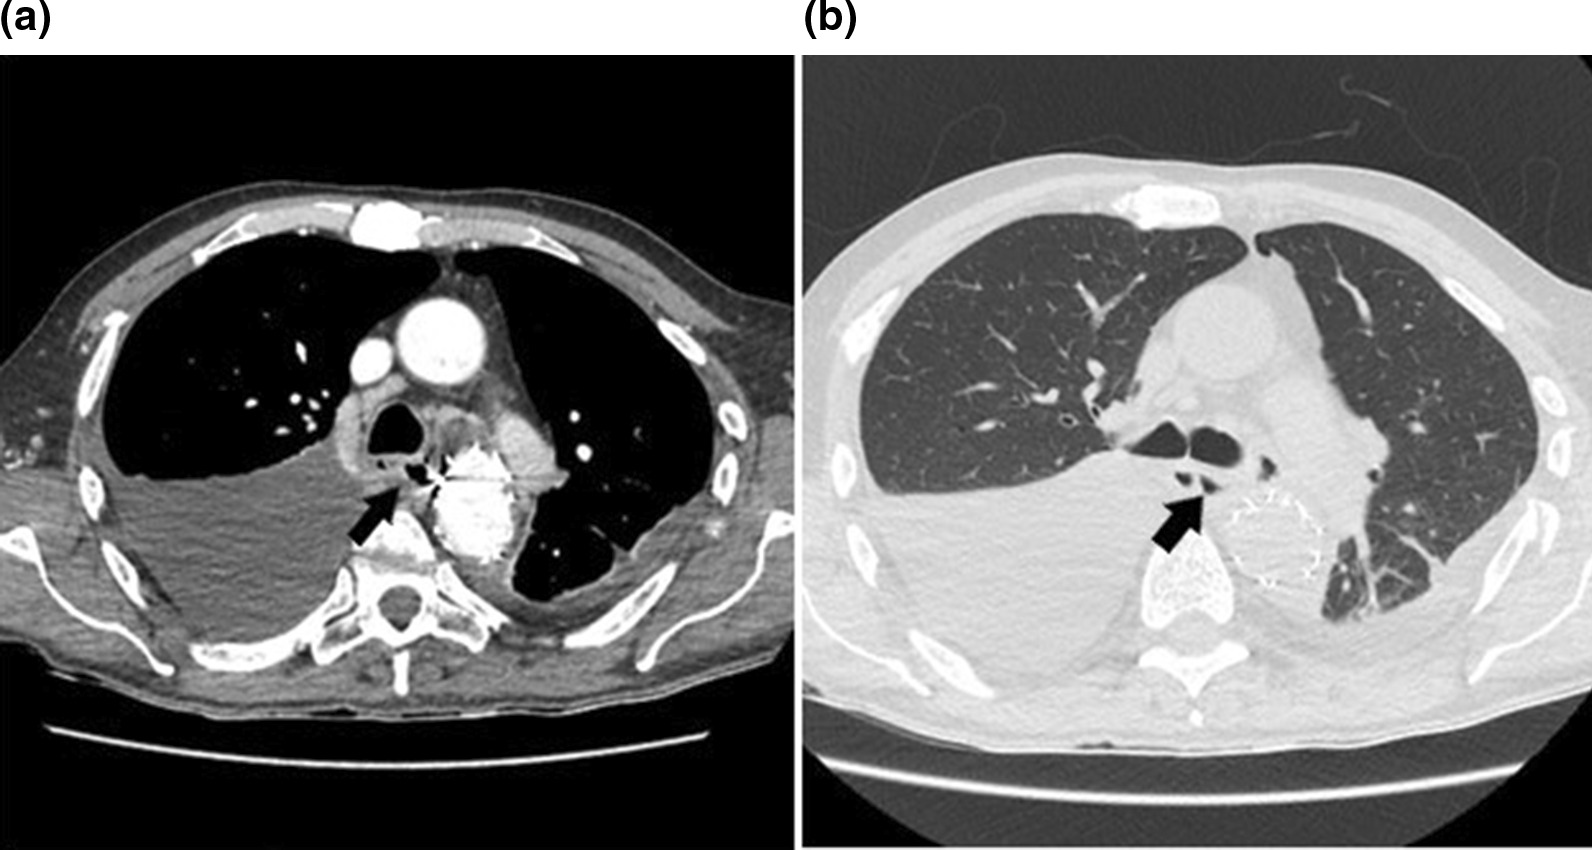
a, b Axial images of CT scan with intravenous contrast, demonstrating normal positioned aortic stent graft, bilateral pleural effusions and pneumomediastinum, indirect sign of esophageal perforation
